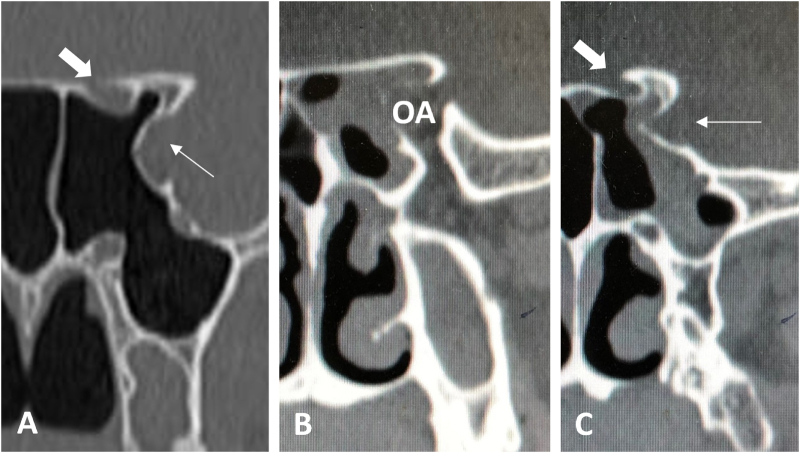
Potential pneumatization pathways of the anterior clinoid process: (A) Axial CT of the left orbital apex showing pneumatized ACP and OS; (B). Pneumatized and involved upper root of the ACP (showing adjacent structure involvement); (C). Pneumatized and involved OS (showing structure involvement). Thick arrows indicate optic canals; thin arrows indicate superior orbital fissures (A, C); OA, orbital apex.
